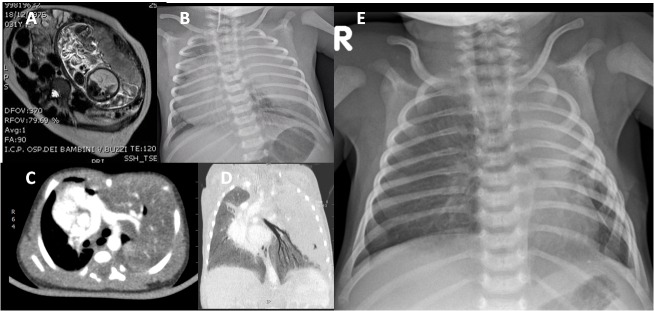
Figure 3: Large left-sided complicated lesion not submitted to fetal therapy for parental refusal: fetal MRI (A), chest X-ray (B) and CT scan (C, D) at birth; the child needed mechanical ventilation at birth and required open surgery by the first 24 hours of life (E: postoperative chest X-Ray).
